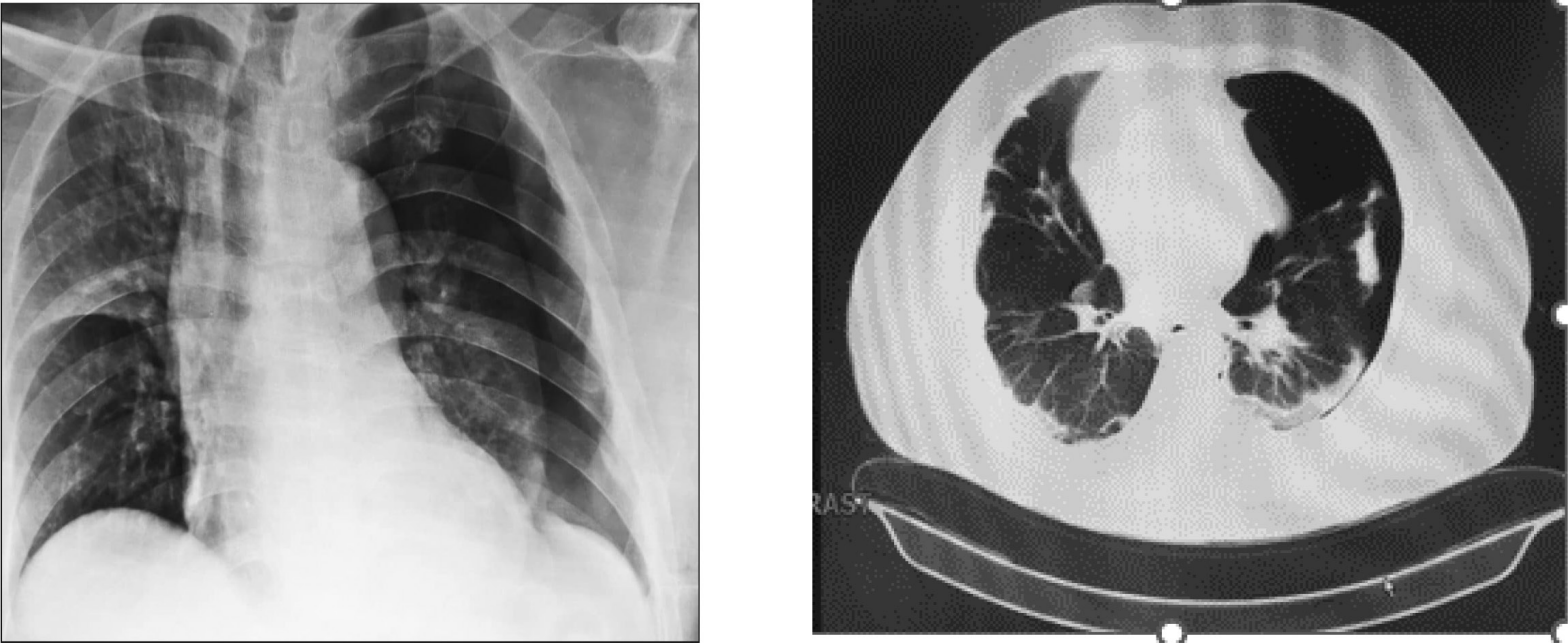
Patient's chest X‐ray and chest CT scan consisting of large left‐sided pneumothorax (early pneumothorax).
